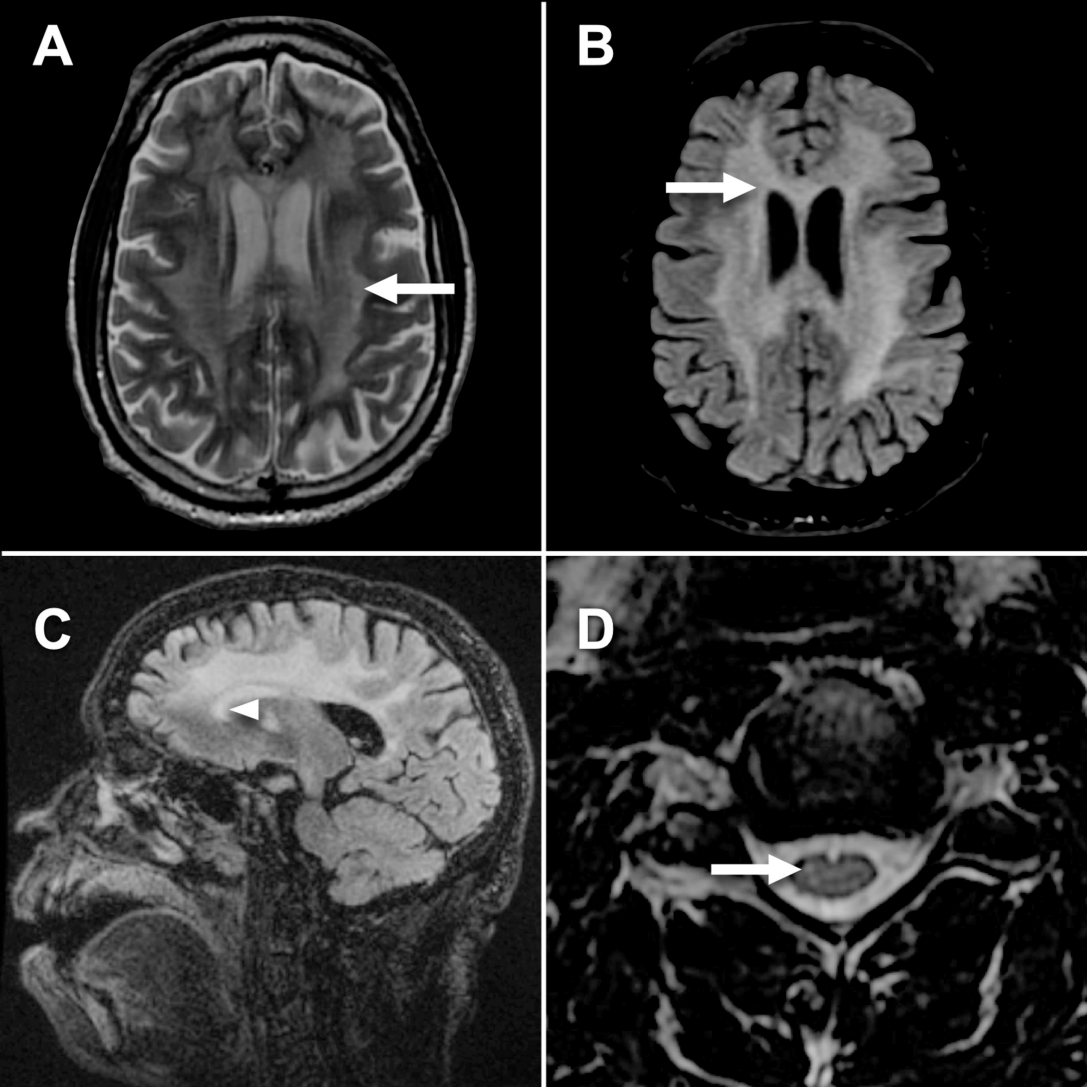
Multiplanar multisequence magnetic resonance (MR) imaging of brain and cervical spinal cord with and without gadolinium contrast. (A) T2 weighted sequence showing increased signal intensity in the white matter in a confluent distribution bilaterally (arrow). (B) T2 fluid attenuated inversion recovery (FLAIR) sequence with increased T2 signal involving same regions as T2 (arrow), but without meningeal signal increase. (C) Sagittal T2 FLAIR sequence showing involvement of the corpus callosum (arrowhead). (D) Axial T2 weighted sequence at the C5 spinal cord level showing small foci of increased T2 signal, suggesting regions of inflammation or demyelination (arrow).
